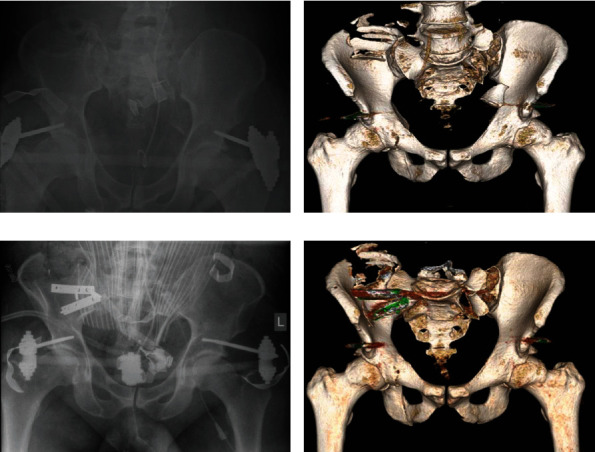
(a) Anteroposterior X-ray of the pelvis at time of administration. (b) 3D-CT reconstruction indicating the instability of the dorsal pelvic ring with a gaping right SI joint. (c) Postoperative anteroposterior X-ray and corresponding 3D-CT reconstruction (d) after plating of the right SI joint and modification of the supracetabular external fixator.
